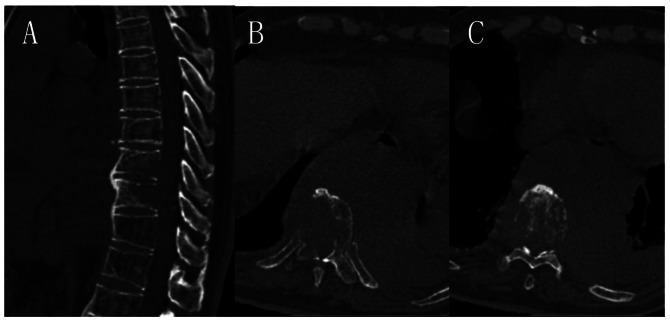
The three-dimensional computed tomography (CT) from T5 to T12. (A) The posterior wall of T9 and T10 was eroded by the tumor mass on sagittal images. (B-C) The CT scans showed extensive lytic involvement of vertebral column and pedicles in T9 and T10, respectively.
